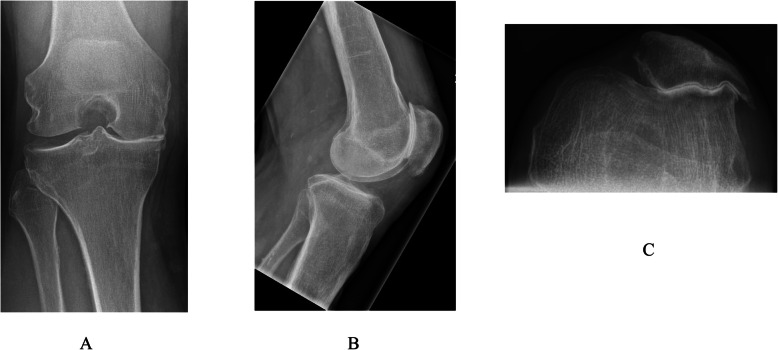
Knee radiographs of ideal candidate for medial segmental bicompartmental knee replacement. a Rosenberg b Lateral c Skyline
